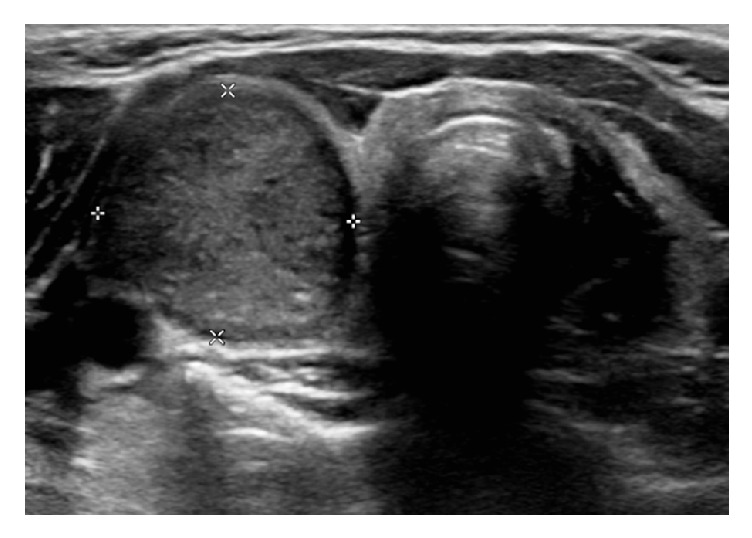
Thyroid ultrasound scan shows a round well circumscribed heterogeneous, highly vascular mass arising from the right lobe of the thyroid, measuring 21 x 17 x 17 mm.
